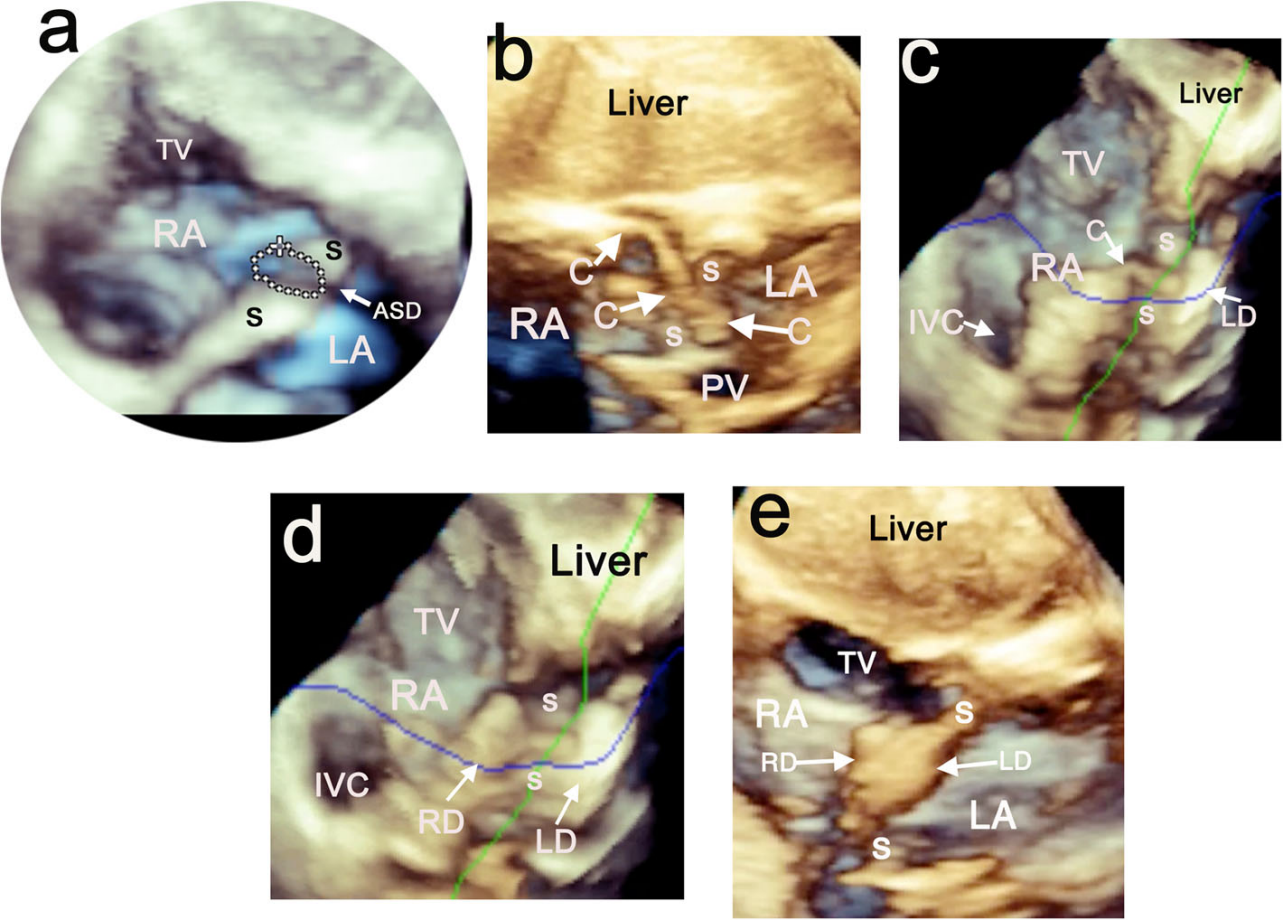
Subcostal views of 3D TTE of MPR images using the AH technique in a 4-year-old boy during ASD closure. a The en face oblique atrial view shows a very thick atrial septum (S) and an ovoid-shaped defect measuring 4 × 8 mm in size. b The entire route of the delivery cable (C) passing correctly from the RA across the atrial septum to the LA. c The LD was deployed in the LA and pushed against the atrial septum (S). d The RD was deployed in the RA. e The occluder was deployed in the approximate position and its final position was confirmed by TEE images. Abbreviations: AH, artificial hand; TTE, transthoracic echocardiography; MPR, multiplanar reformatting; ASD, atrial septal defect; TV, tricuspid valve; C, delivery cable; PV, pulmonary vein; IVC, inferior vena cava; RD, right disc; LD, left disc
